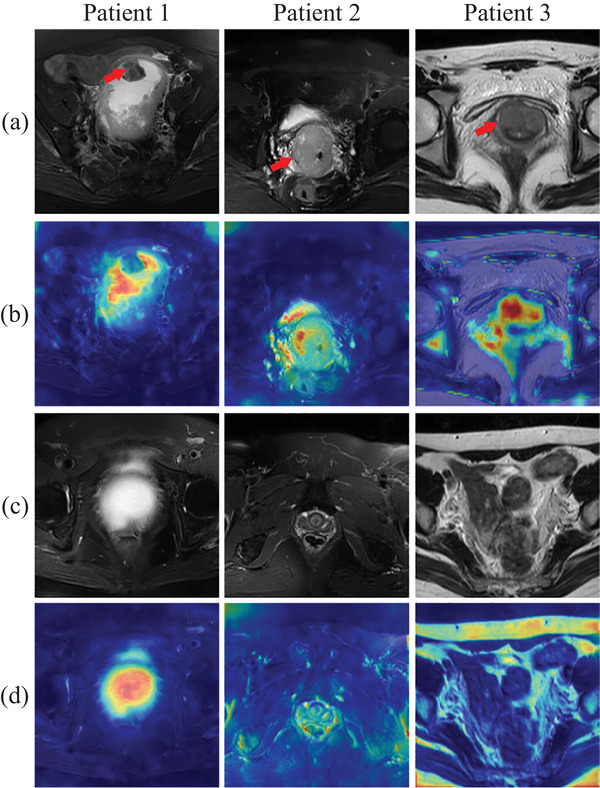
Visualization by using the Grad‐CAM algorithm.^35^ (a) MRI slices with the highest attention scores from three patients; (b) visualization of (a); (c) MRI slices with the lowest attention scores from the same patients in (a); (d): visualization of (c). Red color corresponds to higher gradient values, while blue color corresponds to lower gradient values. Red arrows in (a) point to tumor regions.
